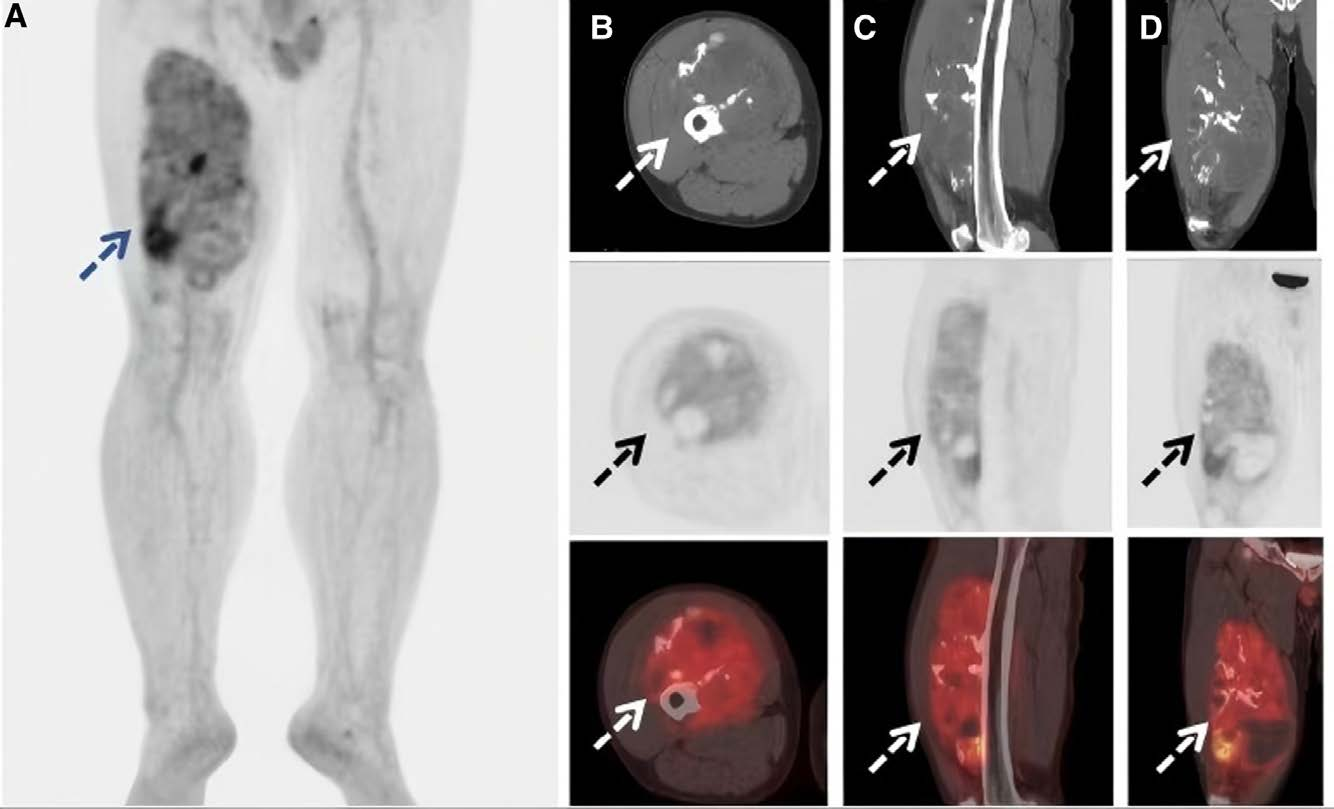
The whole-body MIP image (A) showed increased radiotracer uptake in the soft tissue of right thigh (blue dashed arrow). Right femur images showed a huge area of heterogeneously increased ^18^F-FDG uptake (SUVmax, 4.4) in the right thigh (white and black dashed arrow; B, axial; C, sagittal; D, coronal). 18F-FDG PET/CT = fluorine-18 fluorodeoxyglucose positron emission tomography/computed tomography, MIP = maximum intensity projection.
